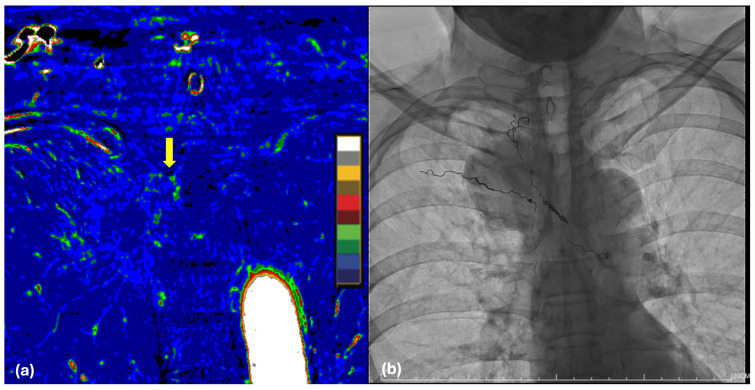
Preoperative hemostatic optimization and vascular assessment. (a) DECT demonstrating moderate hypervascularity within tumor (arrow). (b) Post-procedural radiograph following TAE showing the successful placement of embolization coils within the T3 and T4 intercostal.
